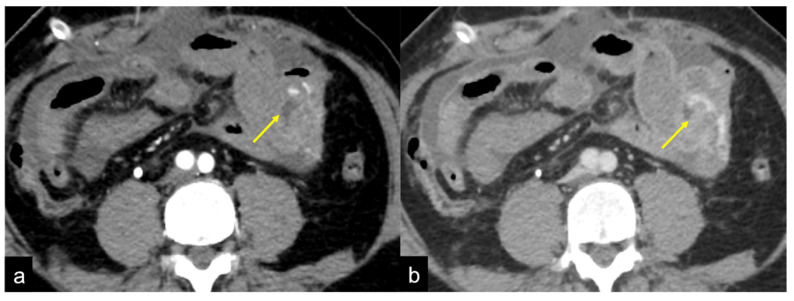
CT multiphasic study of active bleeding in the jejunum. In the arterial phase (a), a blush of active bleeding is detected (arrow). In the venous phase (b), the increase in haemorrhagic blush is documented with contiguous small bowel loop involvement (arrow).
